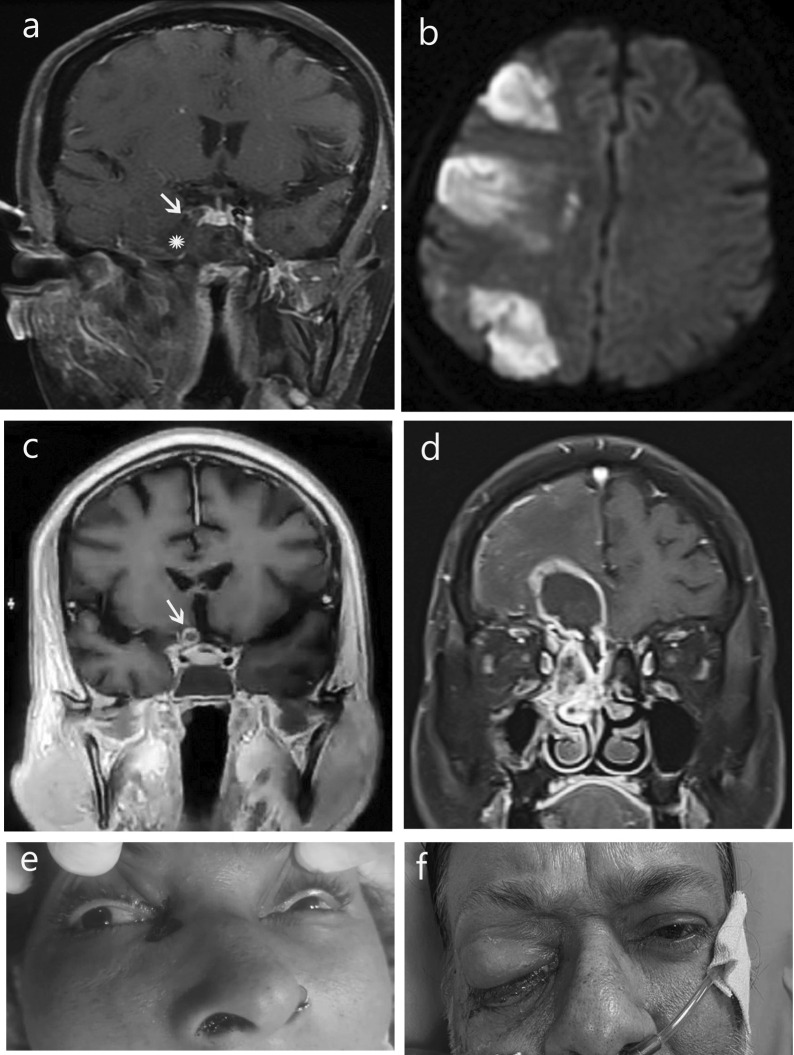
Intracerebral and vascular complications. a Non-enhancing soft tissue replacing right cavernous sinus (*) with loss of flow void in internal carotid artery (arrow), enhancing soft tissue inflammation in temporal and infratemporal fossa. b DWI showing restricted diffusion in multiple water shed infarcts. c Enhancing intracerebral optic nerve (arrow) on right side. d Cribriform plate erosion and ethmoidal mucosal thickening with basifrontal abscess. e Eschar at right medial canthus and nasal opening. f Proptosis and chemosis of right upper lid
